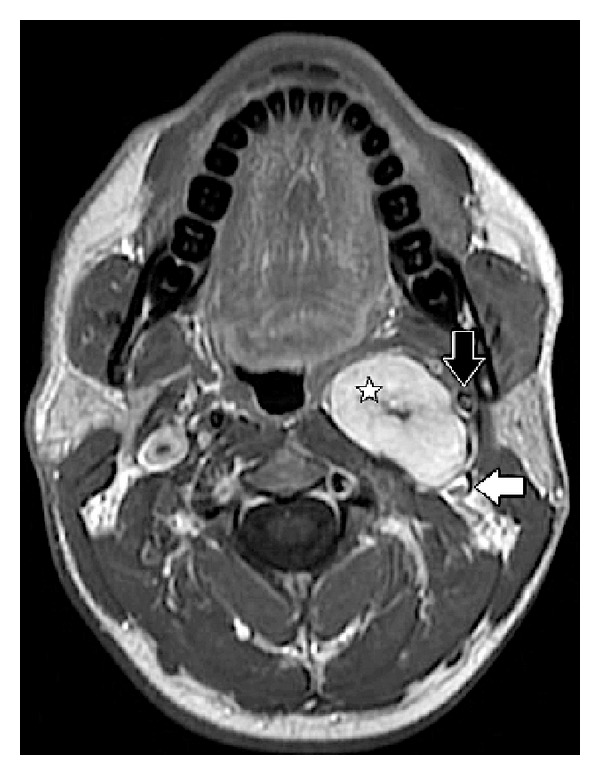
Patient 1 T1-weighted enhanced MRI scan: encapsulated tumor of the left retrostyloid compartment, 5 cm by 4 cm in size (white star). The internal carotid artery (black arrow) is displaced anterolaterally; the internal jugular vein is displaced posteriorly (horizontal white arrow).
